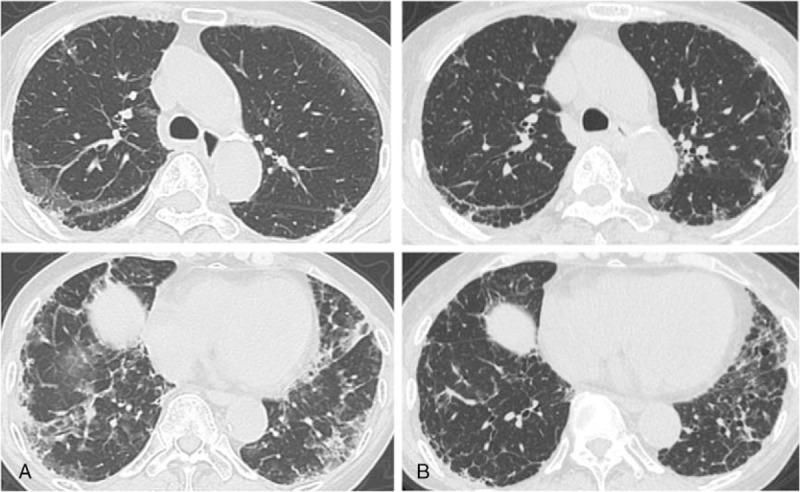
A chest CT on admission showed the expression of invasive shadows on the lung field under the pleura and on the dorsal side of the bilateral lower lobes (A), after the initiation of PE, the invasive shadows showed improvement (B). CT = computed tomography, PE = plasma exchange.
